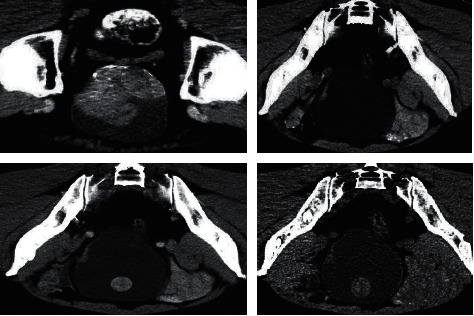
The CT image of a 57-year-old male patient, with a history of high blood pressure.
